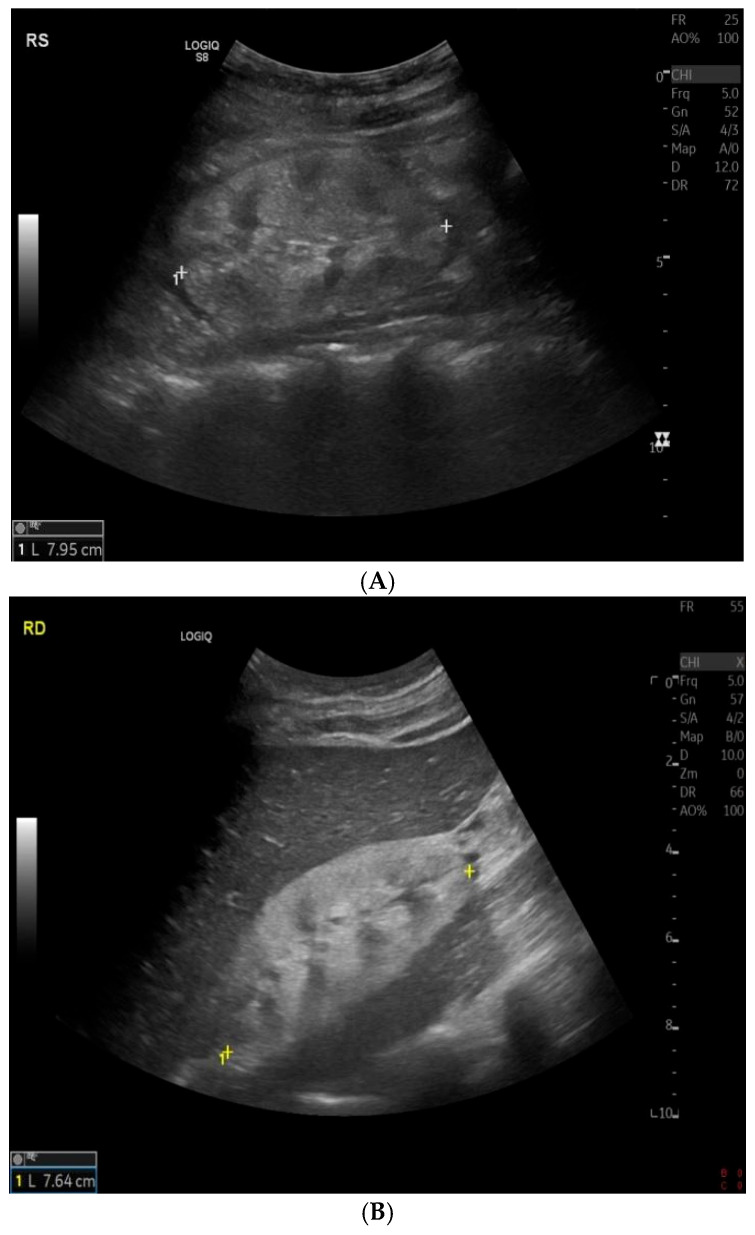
Kidney ultrasound: (A) right kidney; (B) left kidney—both kidneys have reduced diameters and decreased cortico-medullary differentiation.
